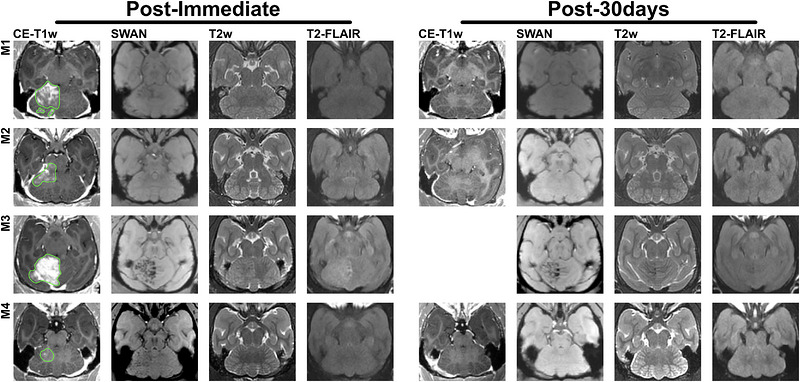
Immediate and 30‐day follow‐up assessment of blood–brain barrier (BBB) opening in the cerebellum. MRI sequences included contrast‐enhanced T1‐weighted imaging (CE‐T1w) with gadolinium (Gd), susceptibility‐weighted imaging (SWAN), T2*‐weighted imaging (T2‐w), and fluid‐attenuated inversion recovery (T2‐FLAIR). Images are shown in axial view at the slice corresponding to the maximal extent of BBB disruption, as indicated by Gd extravasation for all subjects (M1–M4). CE‐T1w for M3 is not available. Imaging was performed immediately after treatment (Post‐immediate) and at 30‐day follow‐up (Post‐30 days).
